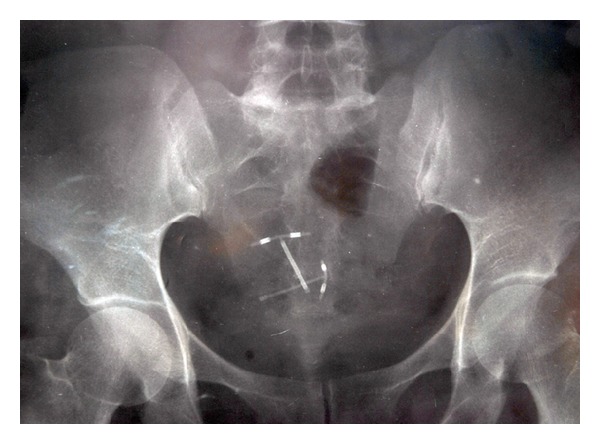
Plain pelvic X-ray showing two copper-T devices.
